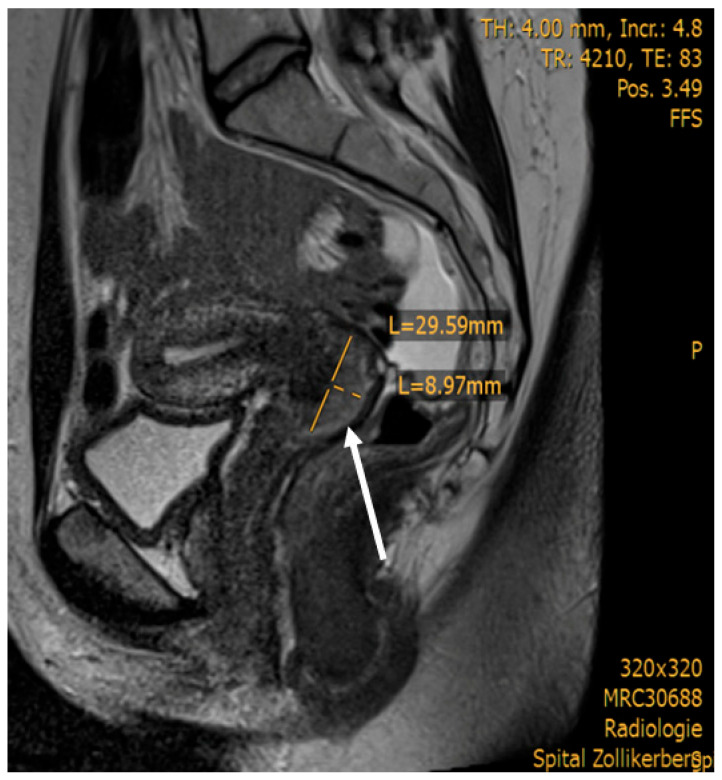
Sagittal MRI demonstrating tumor extent. Cervical carcinoma measuring 30 × 9 mm, located along the dorsal circumference of the cervix uteri, extending from the 9 o’clock to 4 o’clock positions (white arrow).
